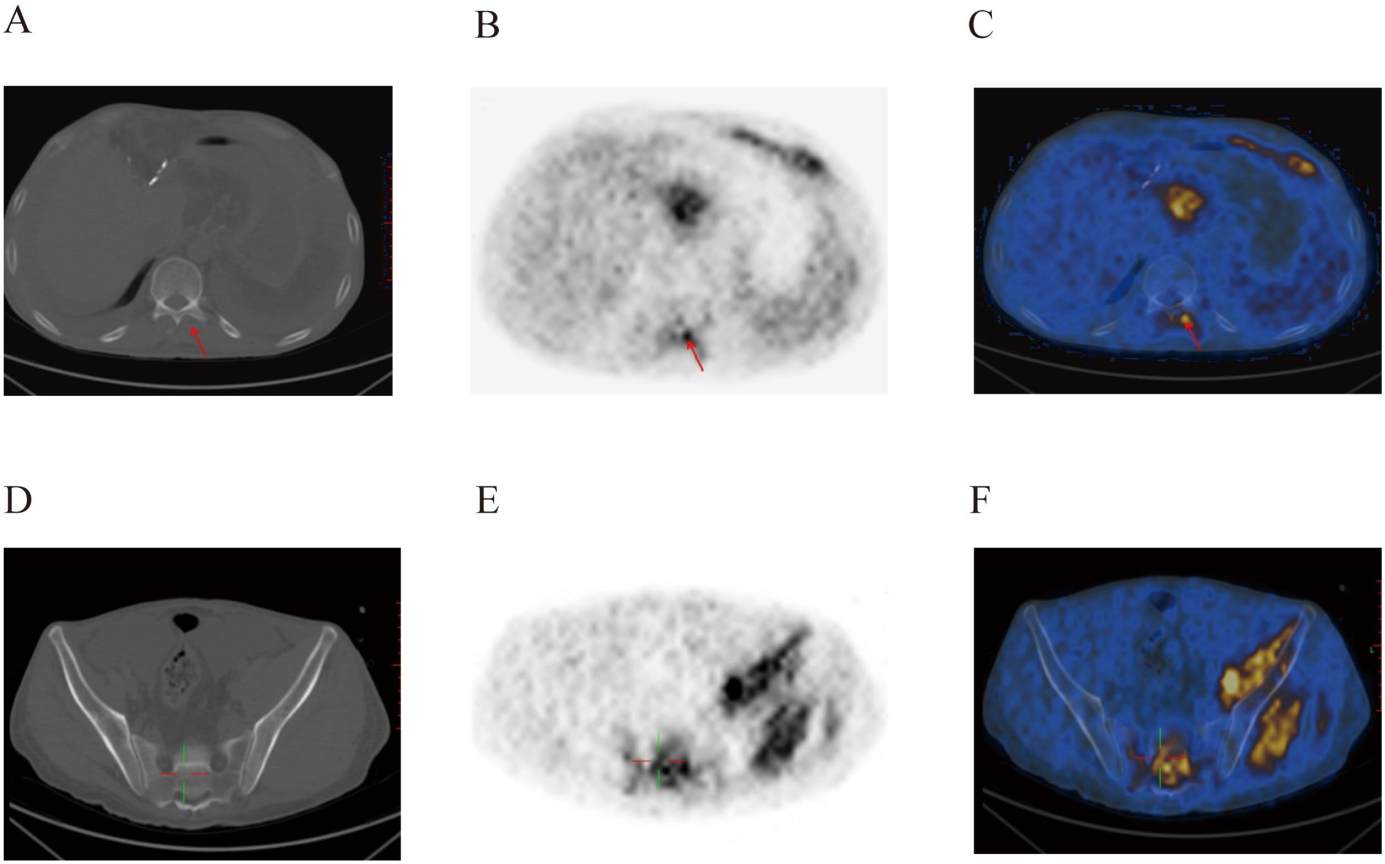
Postoperative PET-CT images of the patient’s spinal canal (A–C) and pelvic region (D–F). Metabolic abnormalities were evident within the spinal canal, and in the left hip muscle.
